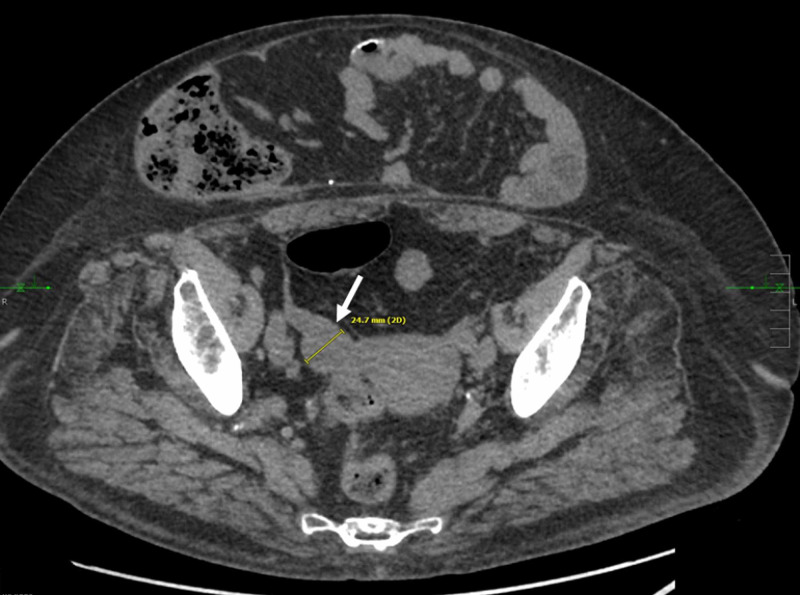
Routine surveillance CT demonstrating no evidence of metastatic disease of the right ovary Axial non-contrast CT obtained 36 months before ablation demonstrates normal right adnexal structures with a right ovary measuring approximately 2.5 cm.
